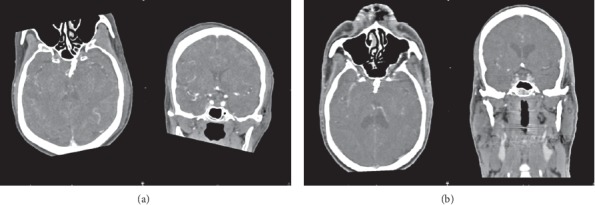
DE-CTA and SE-CTA. Axial and coronal DE-CTA (a) and SE-CTA (b) kv is 100/Sn140 and mAs is 111 [44].
